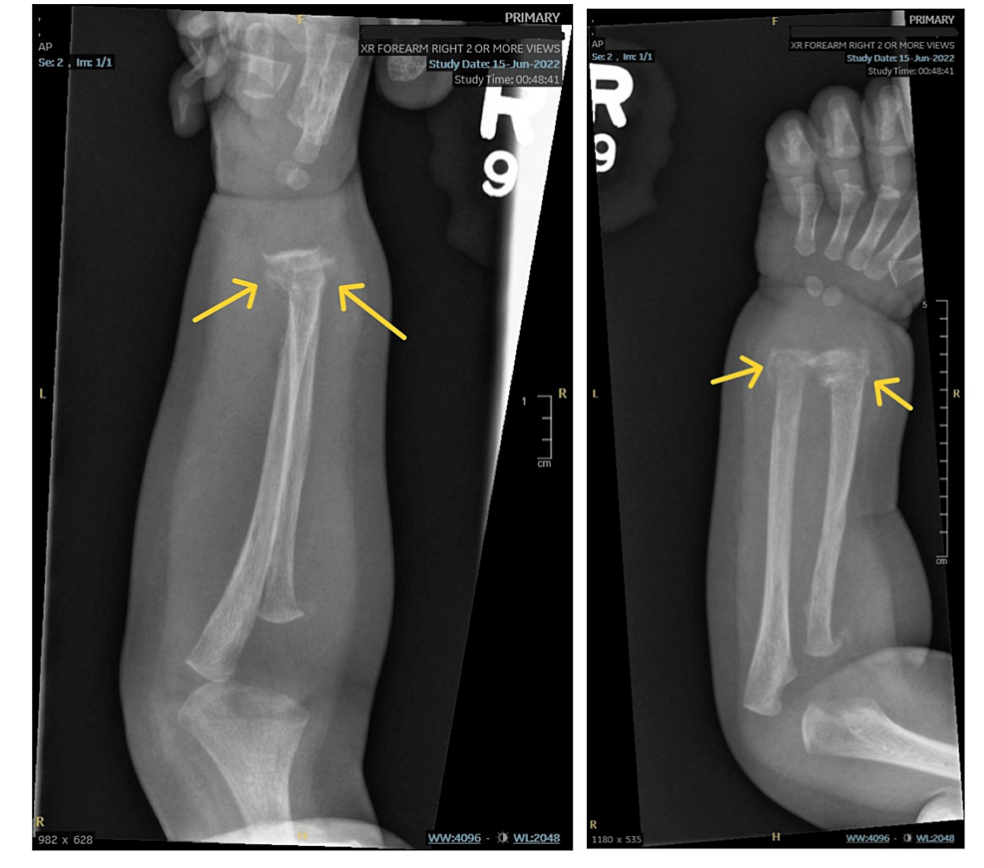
X-rays of healing fractures in the distal radius and ulna on initial presentation
